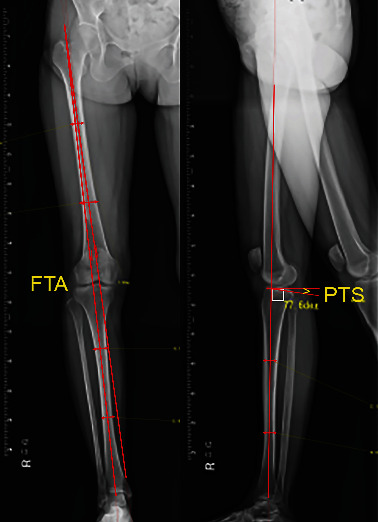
Measurement of the femorotibial angle and the posterior tibial slope angle. The femorotibial angle (FTA) was defined as the lateral angle between the femoral anatomical shaft axis and the tibial anatomical shaft axis on the long leg radiograph at the coronal plane. The posterior tibial slope (PTS) was defined as the posterior angle between the tibial anatomical shaft axis and tibial plateau on the long leg radiograph at the sagittal plane.
